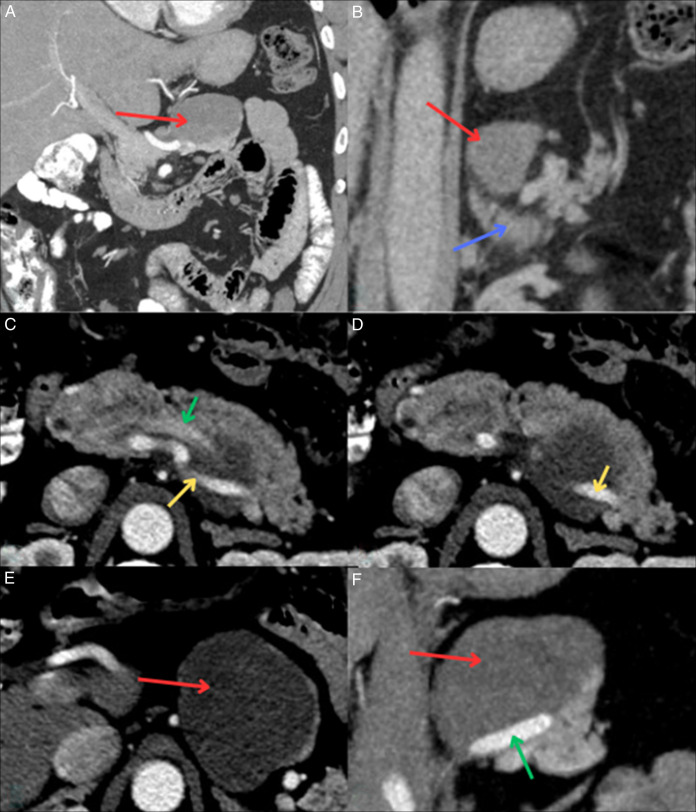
Computed tomography dual pancreas with mass (red arrow) demonstrating no overlap with the left adrenal gland (blue arrow, B) and confirming splenic vessel abutment by the mass on the splenic vein (yellow arrow) and splenic artery (green arrow). A, B, F are coronal views, and C, D, E are axial views. Red arrows also demonstrate mass that is oval and solid in nature with smoother borders, typical of what ganglioneuromas may appear as.
